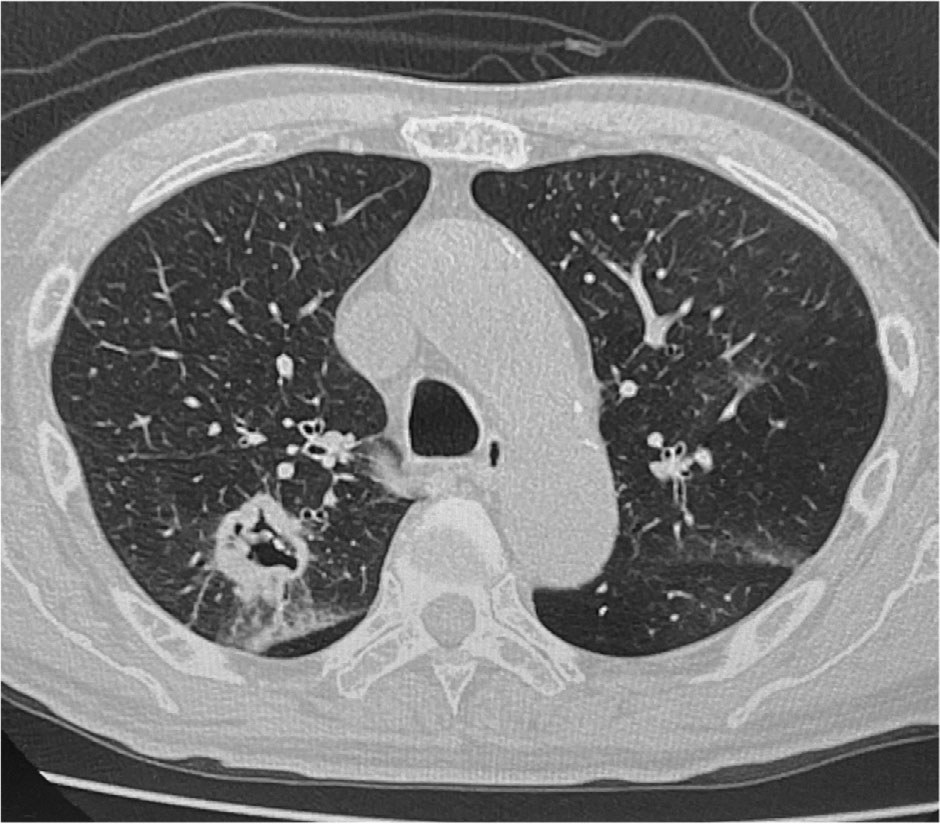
Chest computed tomography showing a 3.4 cm cavitary pulmonary nodule in the right upper lobe as clinical T2aN0M0 stage IB squamous cell carcinoma
